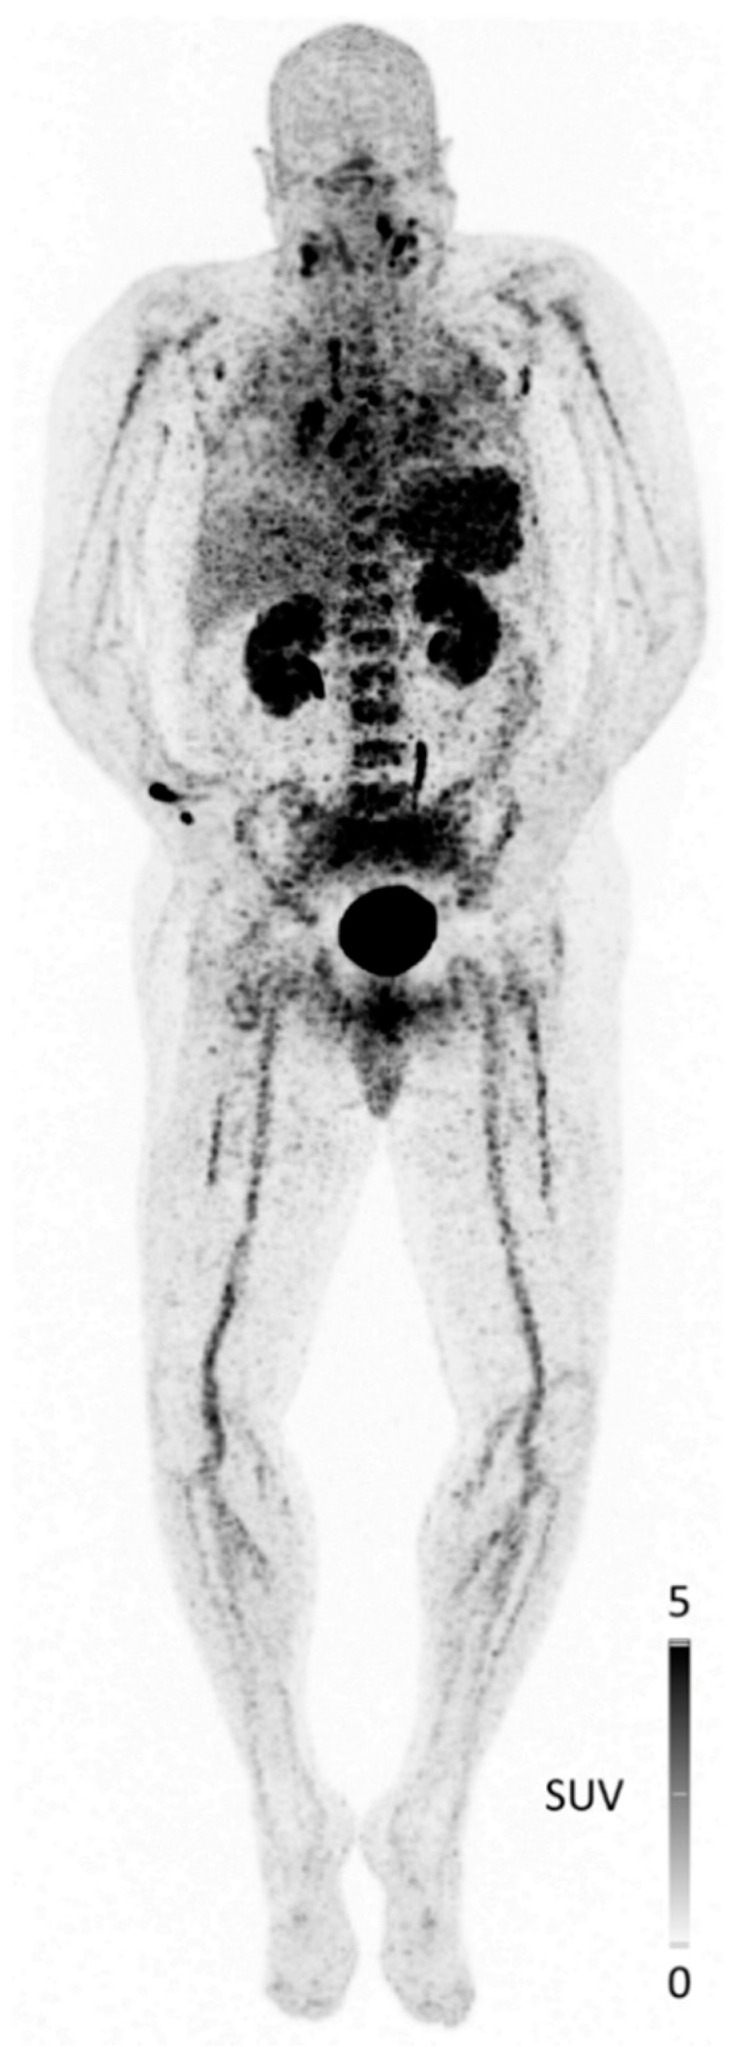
Maximum intensity projection of CXCR4-directed PET/CT with [^68^Ga]Pentixafor in a 67-year-old patient with acute COVID-19 infection. Beyond bilateral pneumonia with reactive hilar and mediastinal lymph nodes, inflammatory foci in the lymphoid tissue of the neck could be depicted. In addition, the reactive activation of both bone marrow and spleen is visualized. The patient’s condition deteriorated quickly after imaging and he was transferred to the ICU on the day after PET/CT [C. Lapa, unpublished data].
